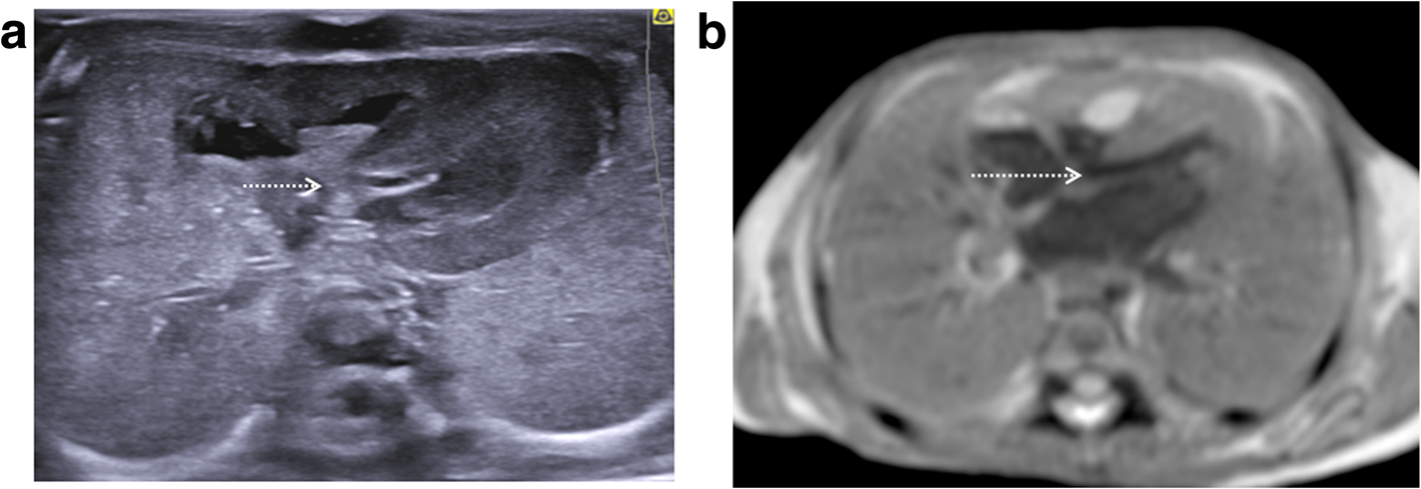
Paired transverse images of the four cardiac chambers in a foetus at 19 weeks gestation, obtained 6 days after termination of pregnancy. Post-mortem ultrasound image (a) and T2-weighted MRI at 1.5T (b) both show a small ventricular septal defect (dotted white arrow). It is also interesting to note the normal ‘expected’ post-mortem intra-cardiac clot (echogenic material on ultrasound, low signal on MRI) and fluid (hypoechoic on ultrasound, high signal on MRI)
